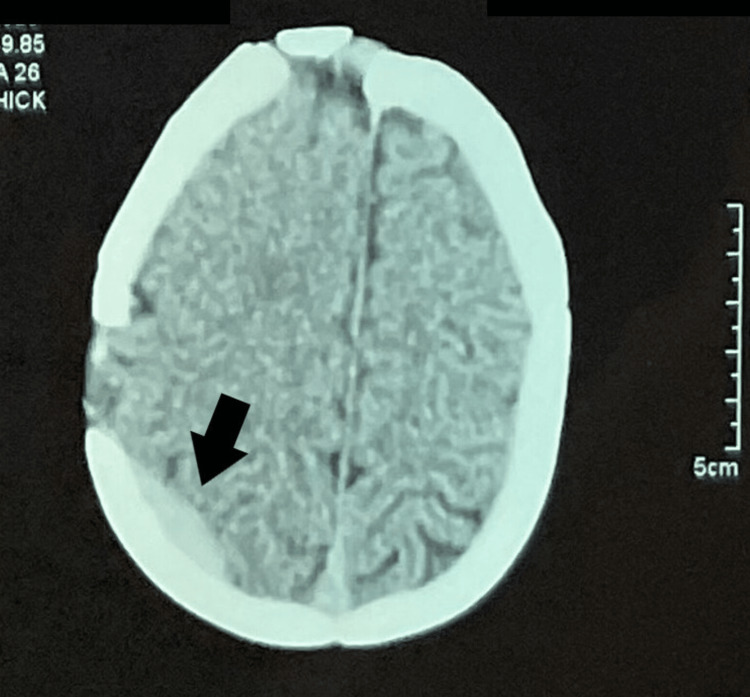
Control CT scan of the head showing a right parietal extradural hematoma (black arrow).
